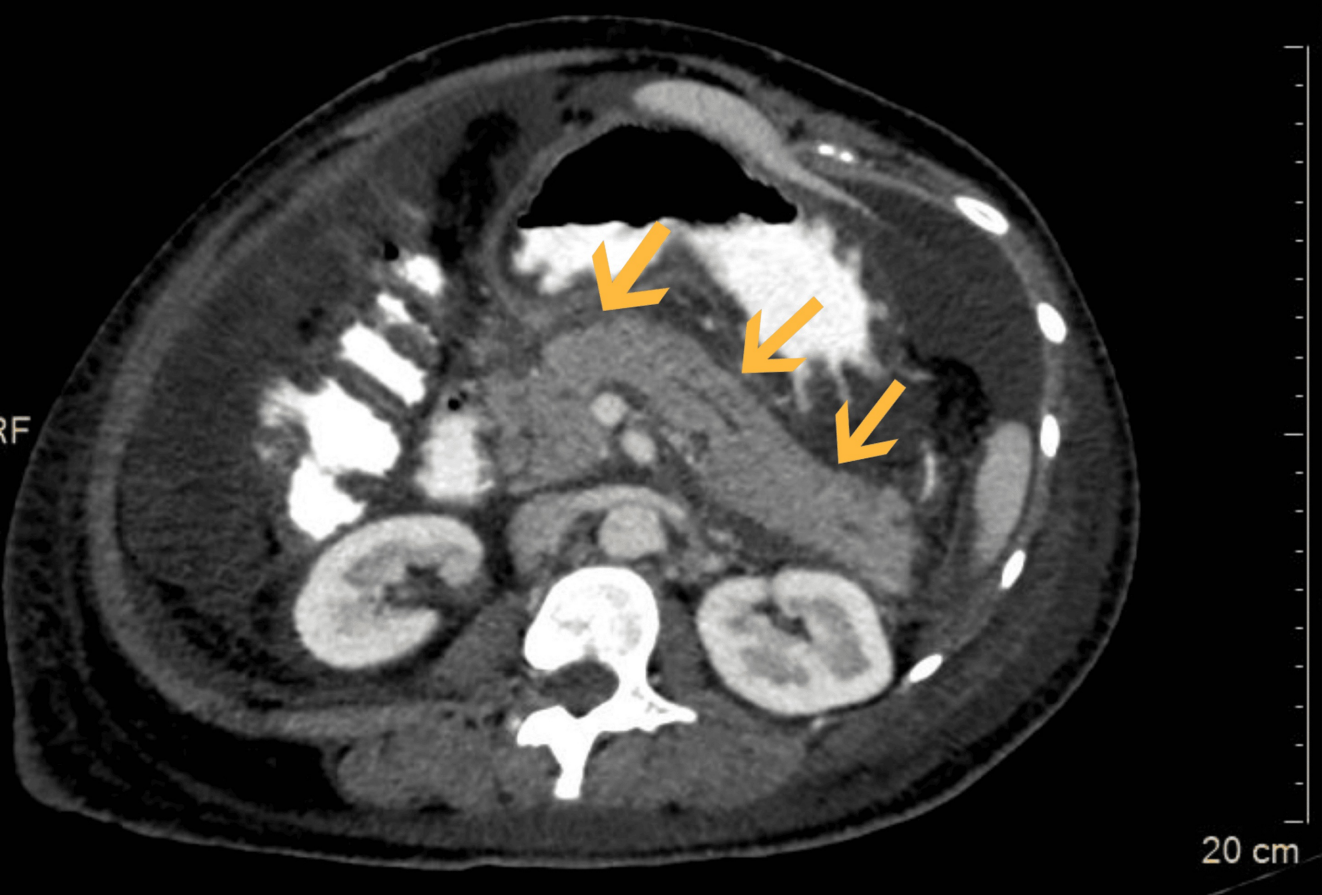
Axial sections of contrast-enhanced computed tomography of the abdomen and pelvis Contrast-enhanced computed tomography abdomen axial cut with oral contrast seen in the portal phase showing a bulky, uniformly, non-enhancing pancreas as indicated by the arrow marks.
